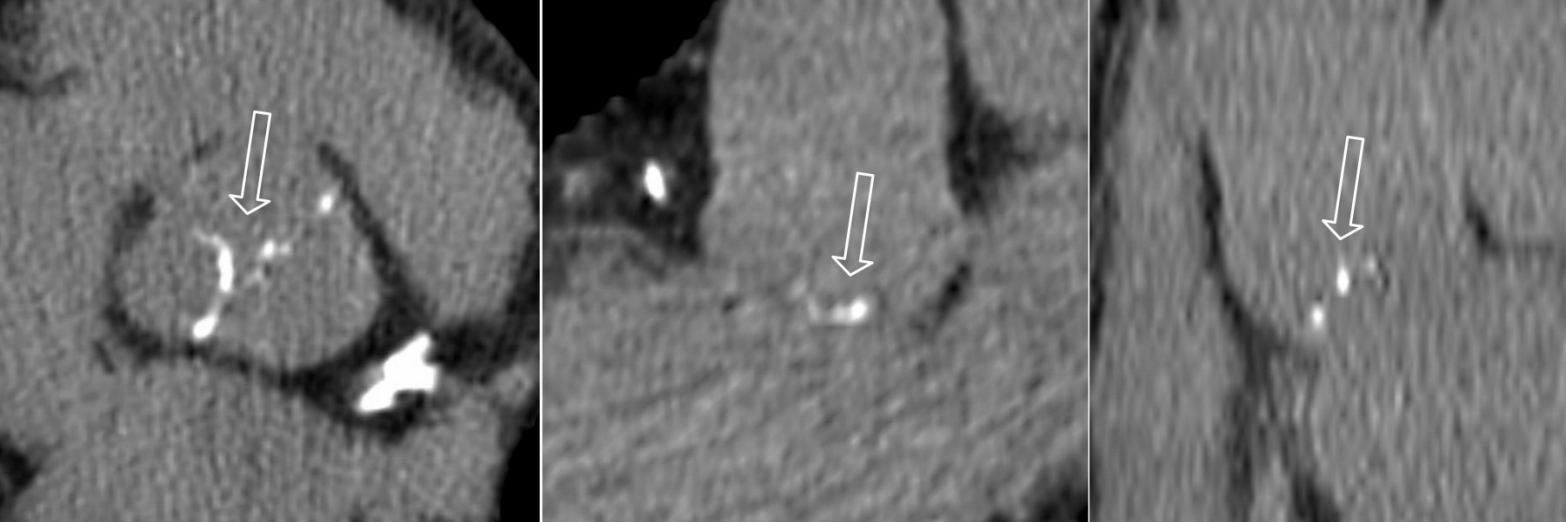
Computed tomography multiplanar refortmats in 3 orthogonal planes confirming the location of the aortic valve calcifications (arrows).
